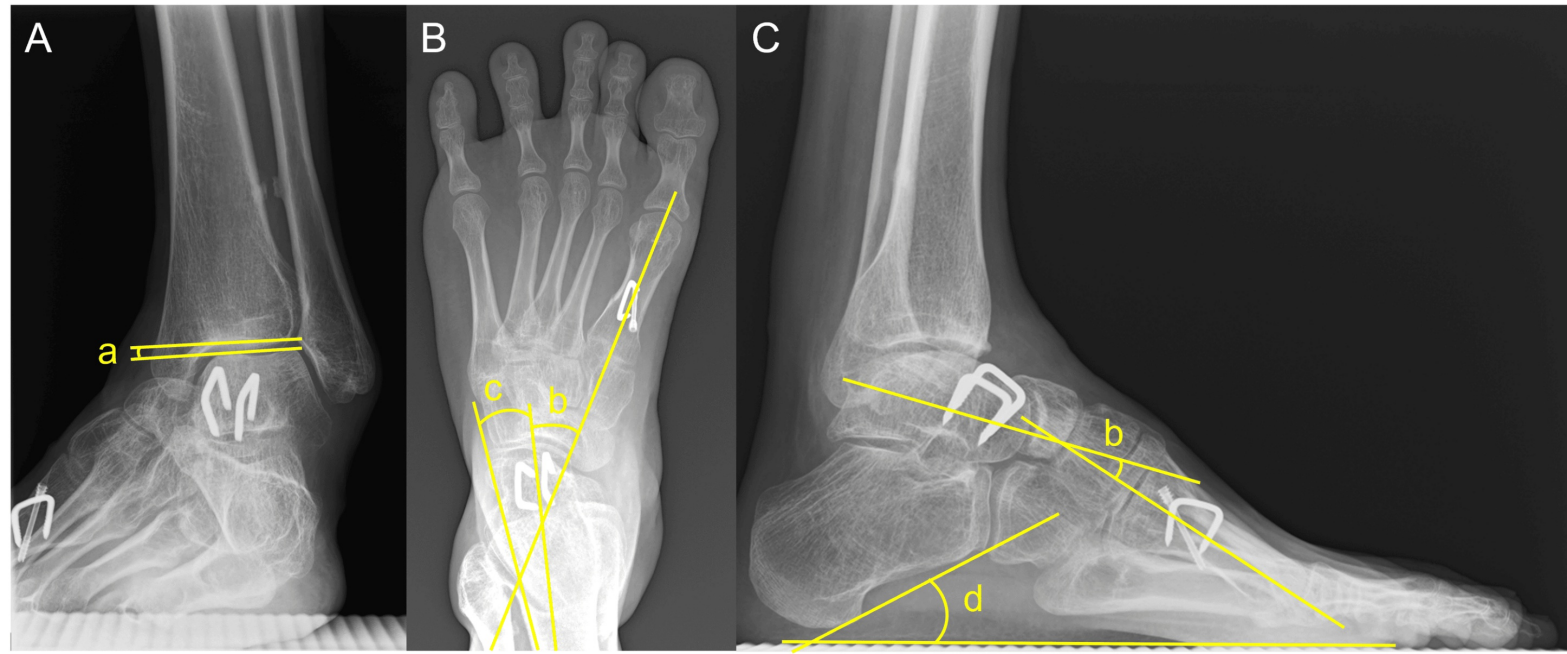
Weight-bearing radiographs at 24 months (A) Anteroposterior view of the ankle; (B) anteroposterior view of the foot; (C) lateral view of the foot. (a) Talar tilt angle; (b) talo-first metatarsal angle; (c) talo-calcaneal angle; (d) calcaneal pitch angle
